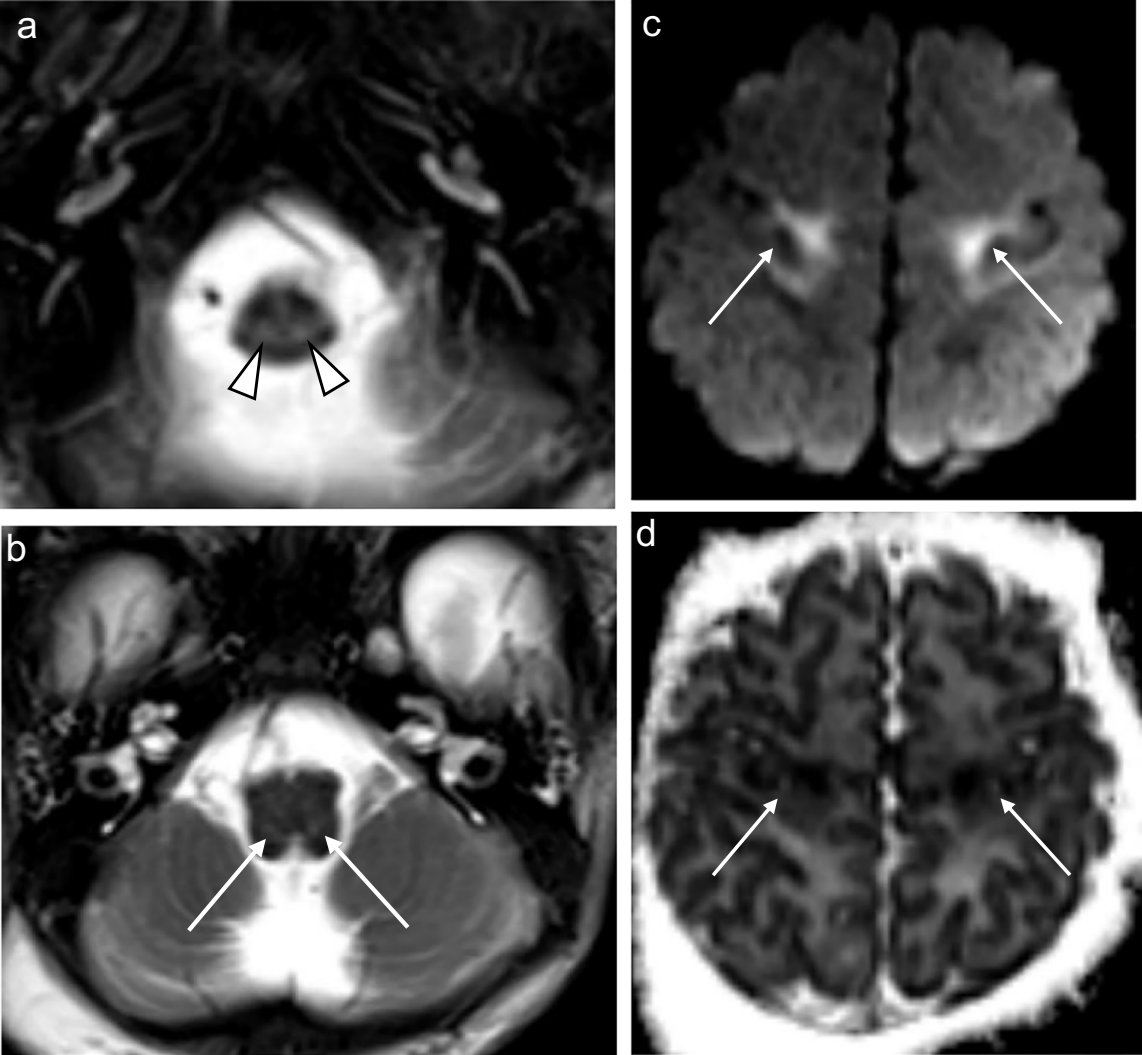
An 11-day-old boy with genetically confirmed *MPV17*-related mitochondrial depletion syndrome. a Axial T2-weighted image shows hyperintense signal in the reticulospinal tracts of the proximal cervical cord (*arrowheads*). b Axial T2-weighted image shows hyperintense signal in the reticular formation in the medulla (*arrows*). c Axial diffusion-weighted image and (d) apparent diffusion coefficient map demonstrate restricted diffusion in the perirolandic cortex (*arrows*)
